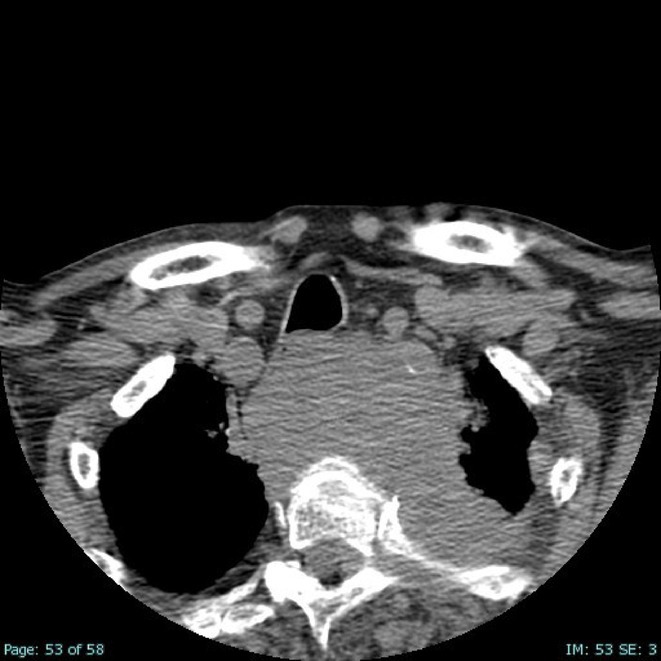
FIGURE 1 Chest CT showing a large solid mass with origin in the left pulmonary apex and invasion of the aorta and mediastinum.
